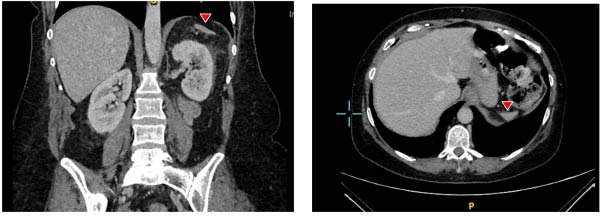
Sagittal CT image showing a markedly atrophic spleen in the left subdiaphragmatic region (red arrow). Retrospective review of a coronal CT scan performed 1 year earlier demonstrated similar splenic atrophy.
